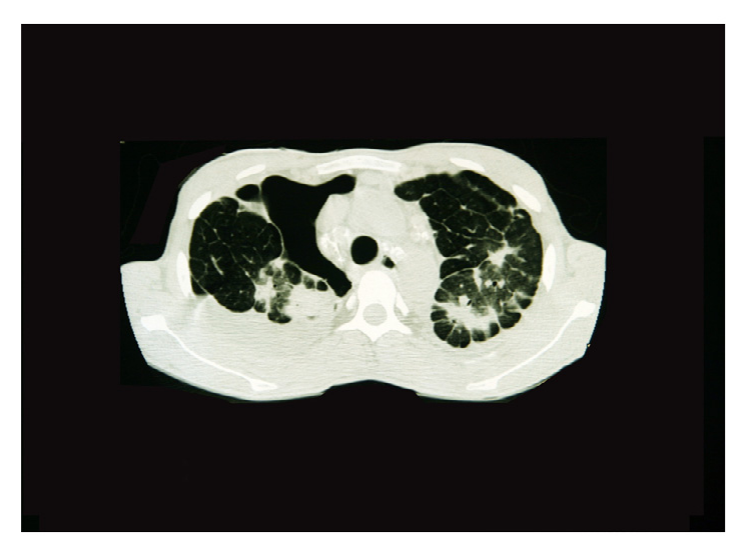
CT. 1: Hydropneumothorax in right lung. 2: Multiple bullae in both lungs. 3: Paraseptal emphysema in left lung. 4: Mixed alveolar and interstitial pattern as silicoproteinosis and fibrosis. 5: Air bronchogram
